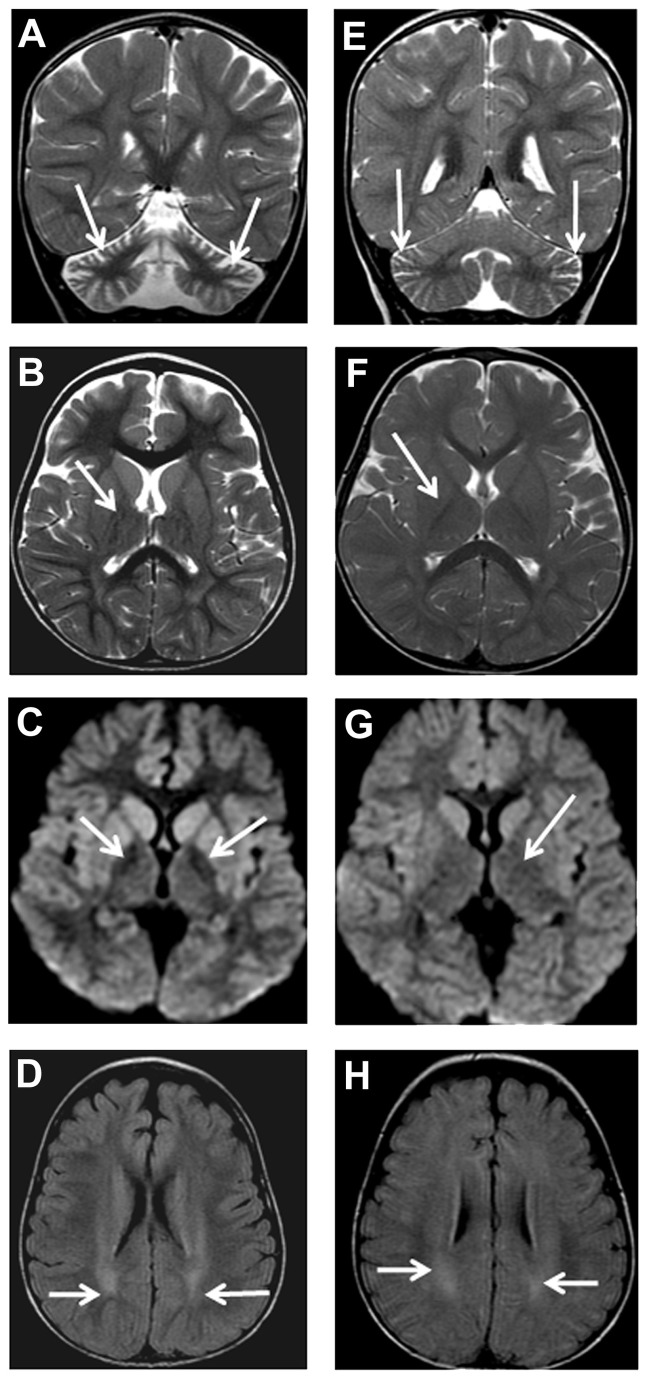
Patient F2 (P1) MRI, age 3 years 3 months (A-D). Coronal T2-weighted (A), axial T2-weighted (B), axial diffusion (C), axial FLAIR (D) showing cerebellar atrophy (arrows) with widening of cerebellar folia (A), T2 normal signal intensity (arrows) of *globus*
